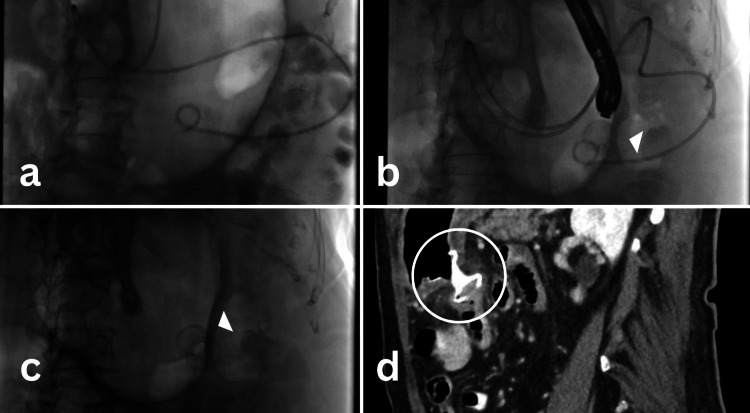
EUS-GE fluoroscopic deployment Images (a-c) and CT image post-procedure (d) (a) The picture shows the placement of the catheter across the stricture into the jejunum. This catheter is used to infuse 500-750 ml of normal saline to distend the small bowel distal to the stricture. (b) The picture shows the placement of LAMS using EUS scope. (c) The picture shows LAMS in place. (d). Post-procedural CT scan showing LAMS in place. EUS-GE: Endoscopic ultrasound-guided gastroenterostomy; LAMS: Lumen apposing metal stent
